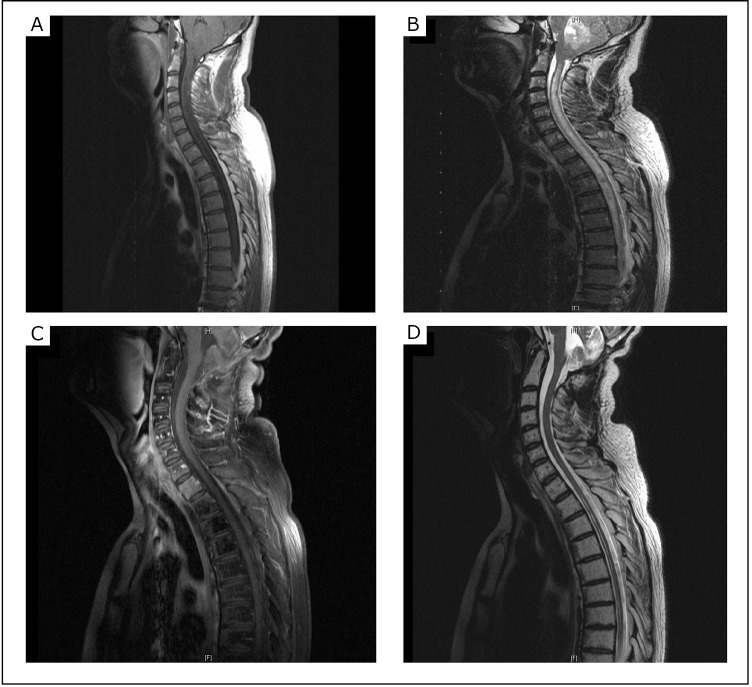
Pre-operative sagittal T1-weighted image with gadolinium enhancement (A) and T2-weighted image (B) showing syringomyelic cavity and no spinal, leptomeningeal spread of tumor. Post-operative sagittal T1-weighted image (C) and T2-weighted image (D) showing the regression of the syrinx.
